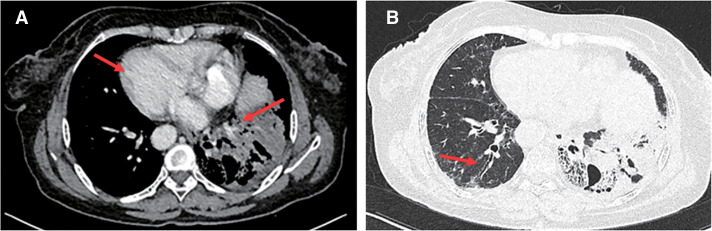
Chest CT (A: mediastinal window, B: lung window). Chest CT revealed chronic inflammation in both lungs, interstitial changes, a mass-like shadow in the left lower lobe (A: the red arrow), partial bronchiectasis (B: the red arrow), and dextrocardia (A: the red arrow). CT: computed tomography
